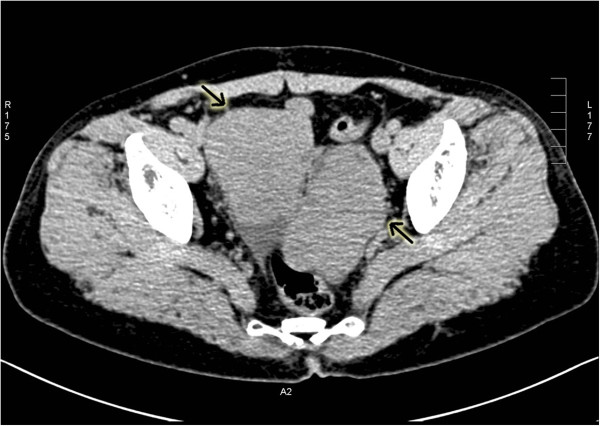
Abdominal CT scans in the patient. Abdominal CT scan was performed in the patient and showed two soft tissue masses in the pelvic cavity (one measuring 7.4×6.4 cm and the other measuring 8.0×4.6 cm). No abnormalities were seen in the bladder, liver, cervical stump or rectum. The arrows indicated the messes.
